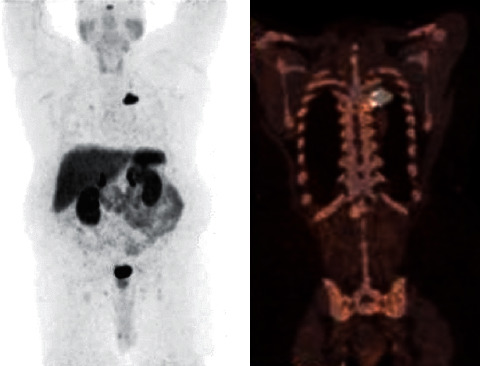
^68^Ga-DOTATATE PET-CT maximum intensity projection coronal (a) and representative-fused PET/CT coronal (b) views demonstrating somatostatin receptor-positive lesion involving the posteromedially located pleural-based 3.5 cm mass in the left upper lobe.
